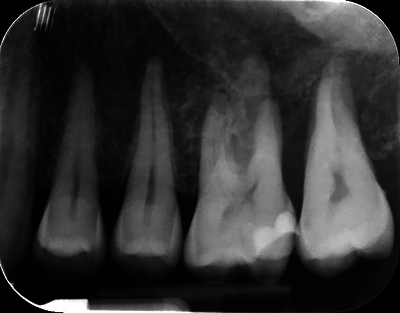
Retro-alveolar radiography at the first appointment.
